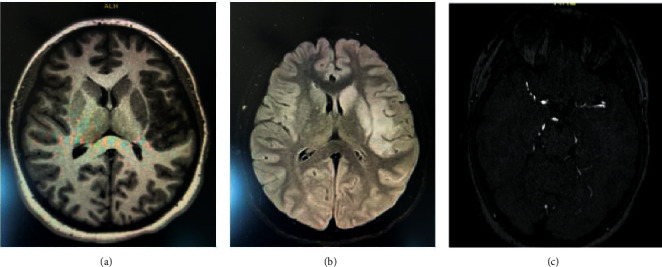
(a) : MRI brain WO contrast. An MRI of the brain showed an acute infarct without hemorrhage involving the left insula and territory of the left middle cerebral artery. (b) MRI brain W-WO contrast. There is a loss of appropriate signal intensity within the M1 segment of the left MCA for a length of appropriate 9 mm. There is a reconstitution of appropriate signal intensity distal to the aforementioned. There is diminutive signal intensity involving the supraclinoid aspect of the right ICA with appropriate reconstitution distally, which may be artifactual in nature as no restricted diffusion is seen within the right cerebral hemisphere. The parent branches, the ACAs, PCAs, basilar artery, and vertebral arteries appear without significant stenosis. (c) MRI angiogram with head WO contrast. There is a demonstration of restricted diffusion involving the left basal ganglia and portions of the left frontal, parietal, and temporal lobes.
